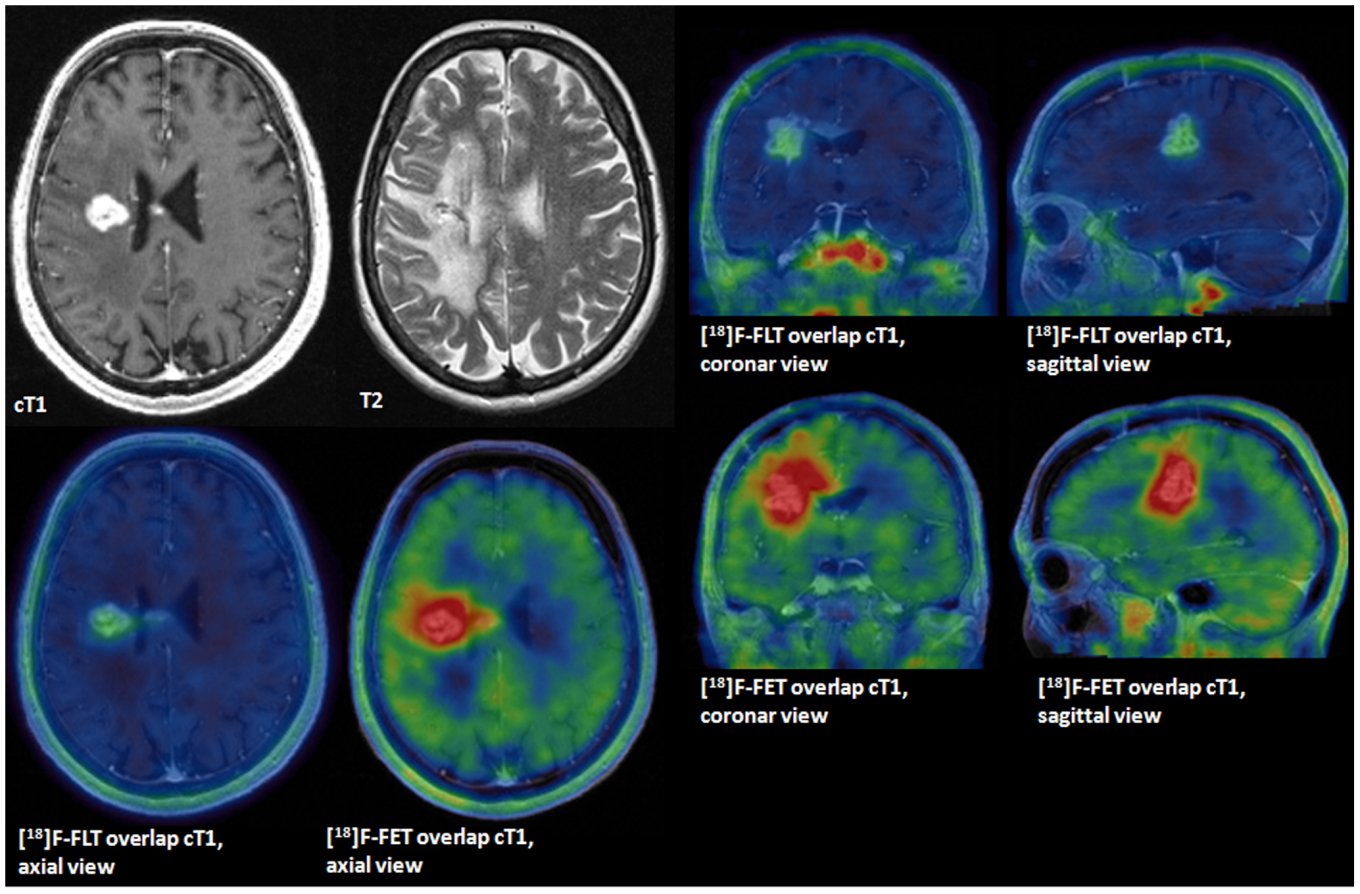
[^18^F]-FET tracer uptake independent from contrast enhancement on MRI, overlap. GBM WHO IV at 2^nd^ tumor recurrence. Contrast enhanced T1 sequences (cT1) tumor volume 5.4 cm^3^, metabolically active tumor volumes for [^18^F]-FET 17.58 cm^3^ and [^18^F]-FLT of 3.9 cm^3^. Overlap analysis detected a [^18^F]-FET volume of 9.2 cm^3^ beyond the borders of cT1.
